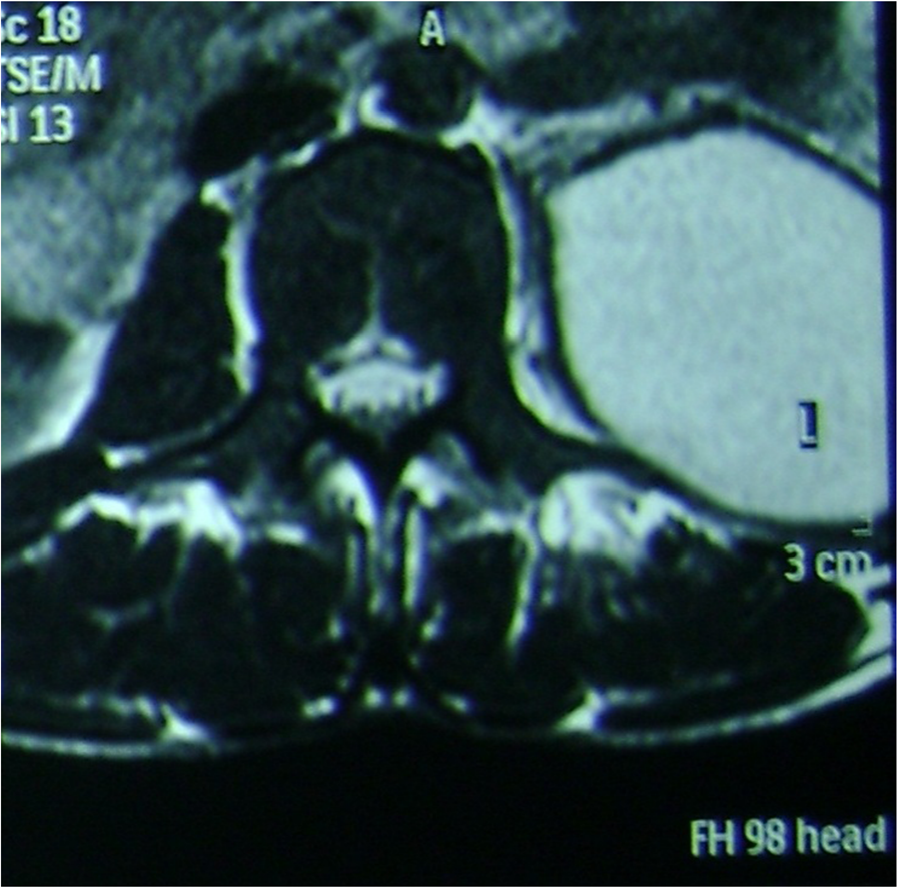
CT scan dorsal spine showing left psoas abscess.
